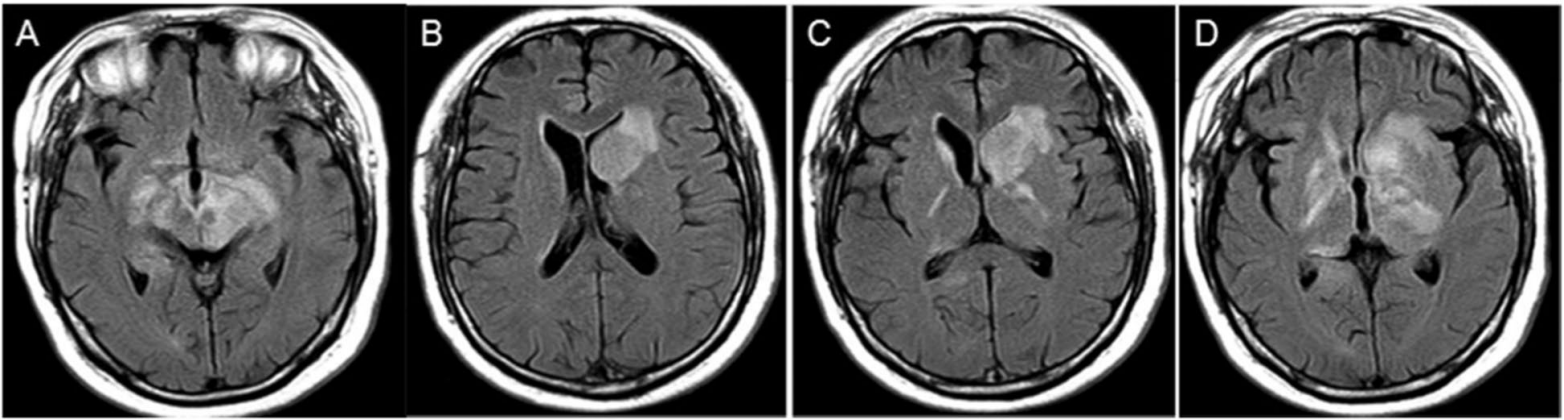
MRI at the second hospital admission. a-d FLAIR weighted, the midbrain and basal ganglia demonstrate hyperintensity, with progressive increase
